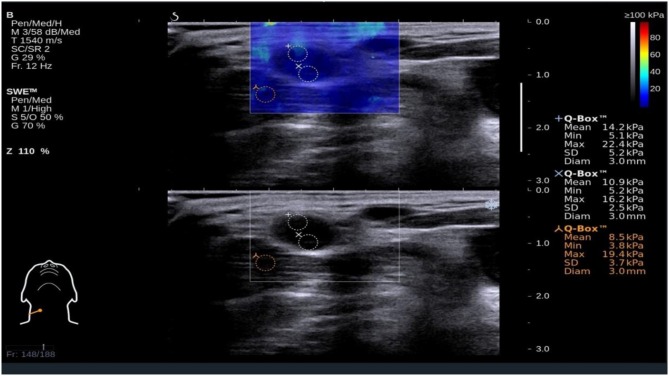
Shear wave elastography evaluation of a benign small cervical lymph nodes (sCLN) biopsy-proven. Shear wave elastography covered the entire target sCLNs and a small amount of surrounding tissue. The shear wave elastography image (up) is presented simultaneously with the gray-scale ultrasound image (below) on the same screen. The node and surrounding tissue exhibited a relatively homogeneous blue color elasticity signal. A 3 mm-diameter Q-box was placed in the node, and the shear elasticity indices of the stiffest region are as follows: Emin (Minimum elasticity values) = 5.2 kPa, Emax (Maximum elasticity values) = 22.4 kPa, and Emean (Mean elasticity values) = 14.2 kPa.
